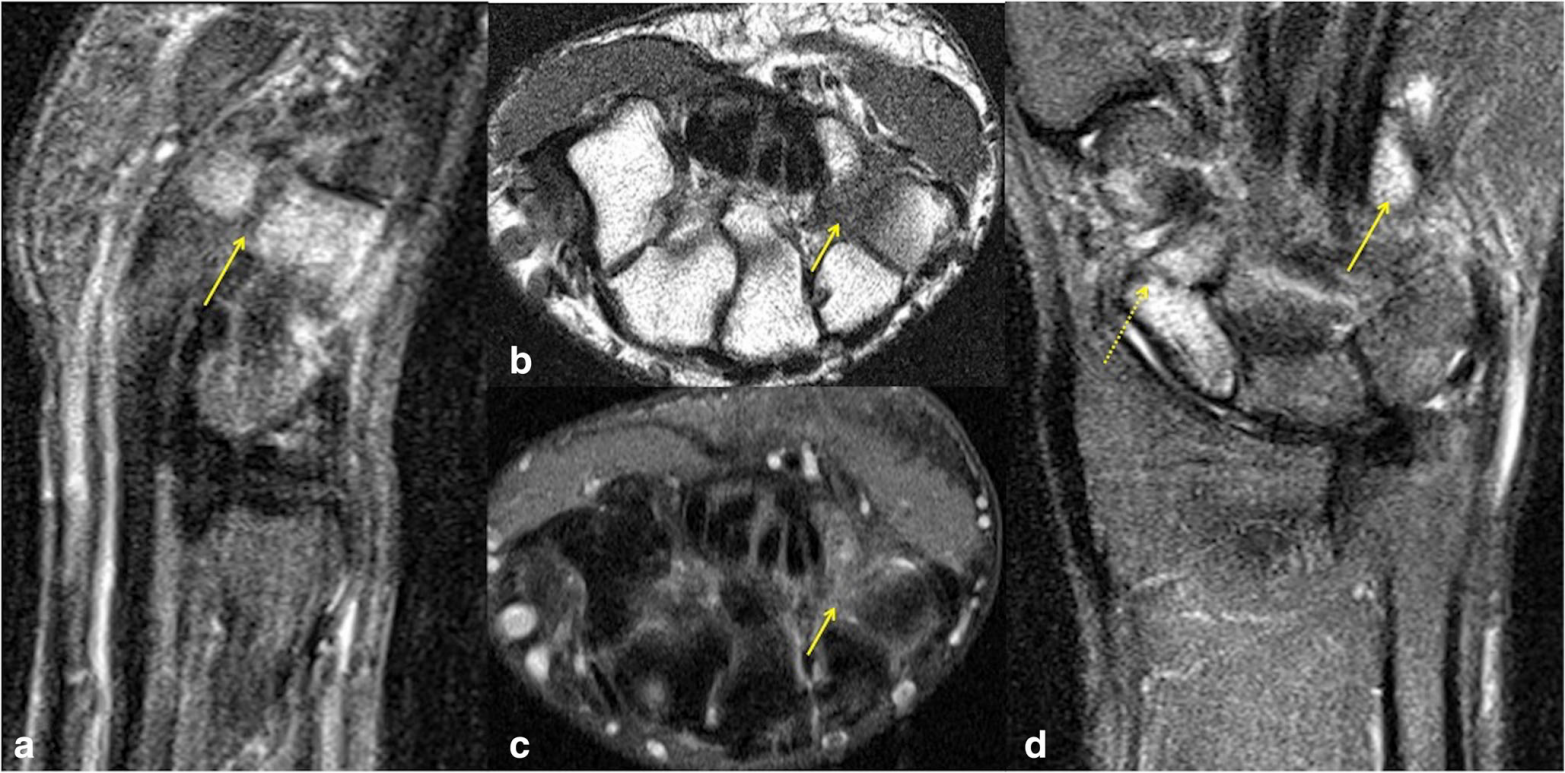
A 21-year-old man with fracture of the left scaphoid and concomitant hook of hamate fracture. a Sagittal STIR shows significant marrow oedema involving the hook and body of the hamate, which is easily discernible compared with the low signal observed in the other imaged bones. A low signal fracture line is seen across the base of the hook of hamate (*arrow*). b Hook of hamate fracture on axial T1 and c corresponding axial STIR sequences, both demonstrating obvious marrow signal abnormality, although the displaced fracture line is more conspicuous on T1. d Coronal STIR sequences easily demonstrate marked oedema with angulated fracture of the distal scaphoid pole (*dashed arrow*), but also draw attention to marked marrow oedema of the hook of hamate (*arrow*). As is generally the rule with MRI of most fractures, bony abnormality was most notable on the fluid-sensitive sequences due to marrow oedema, although the fracture line was most conspicuous on T1
